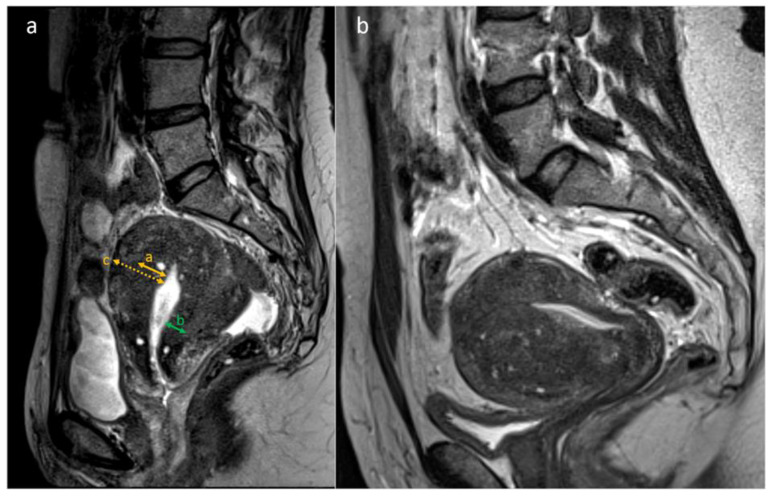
(a,b). MRI features of adenomyosis: T2-weighted sagittal images from two different subjects with adenomyosis. (a). JZmax, the thickest part of the JZ in the midsagittal plane (orange arrow). JZmin, the thinnest part of the JZ in the midsagittal plane (green arrow). Myometrial thickness, measured at the same level as JZmax (orange dotted arrow). (b). Example of a uterus with globular shape and asymmetric thickening of JZ (anterior JZ thicker than posterior JZ). The JZ is irregular due to the presence of multiple hyperintense cystic foci within the thickened JZ.
